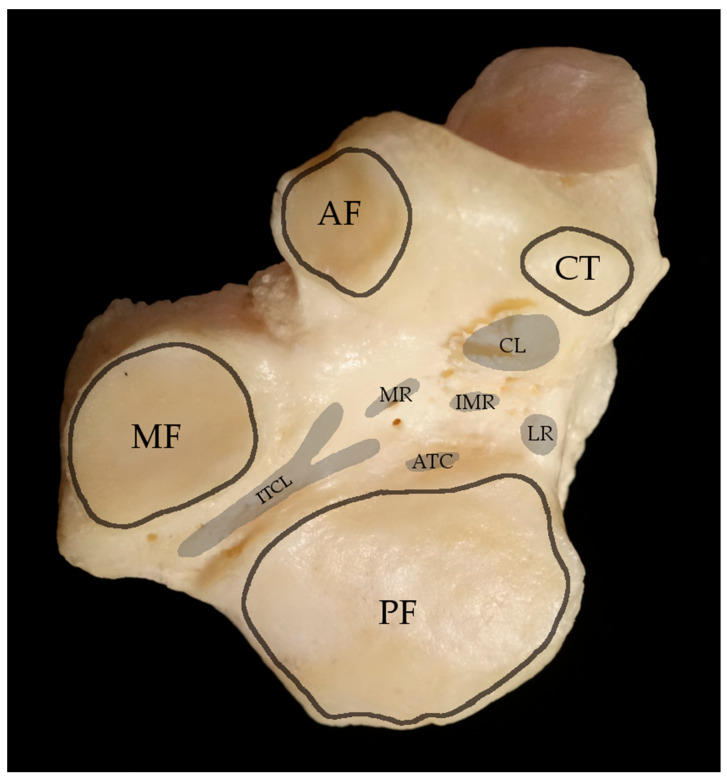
Ligament attachments in the tarsal sinus and tarsal canal. Dorsal view of a right calcaneus. AF = anterior facet, MF = medial facet, PF = posterior facet, CT = crista lateralis. The footprints of the cervical ligament (CL), interosseous talocalcaneal ligament (ITCL), anterior talocalcaneal ligament (ATC) and the roots of the inferior extensor retinaculum are highlighted in grey. MR = medial root, IMR = intermediate root, LR = lateral root.
